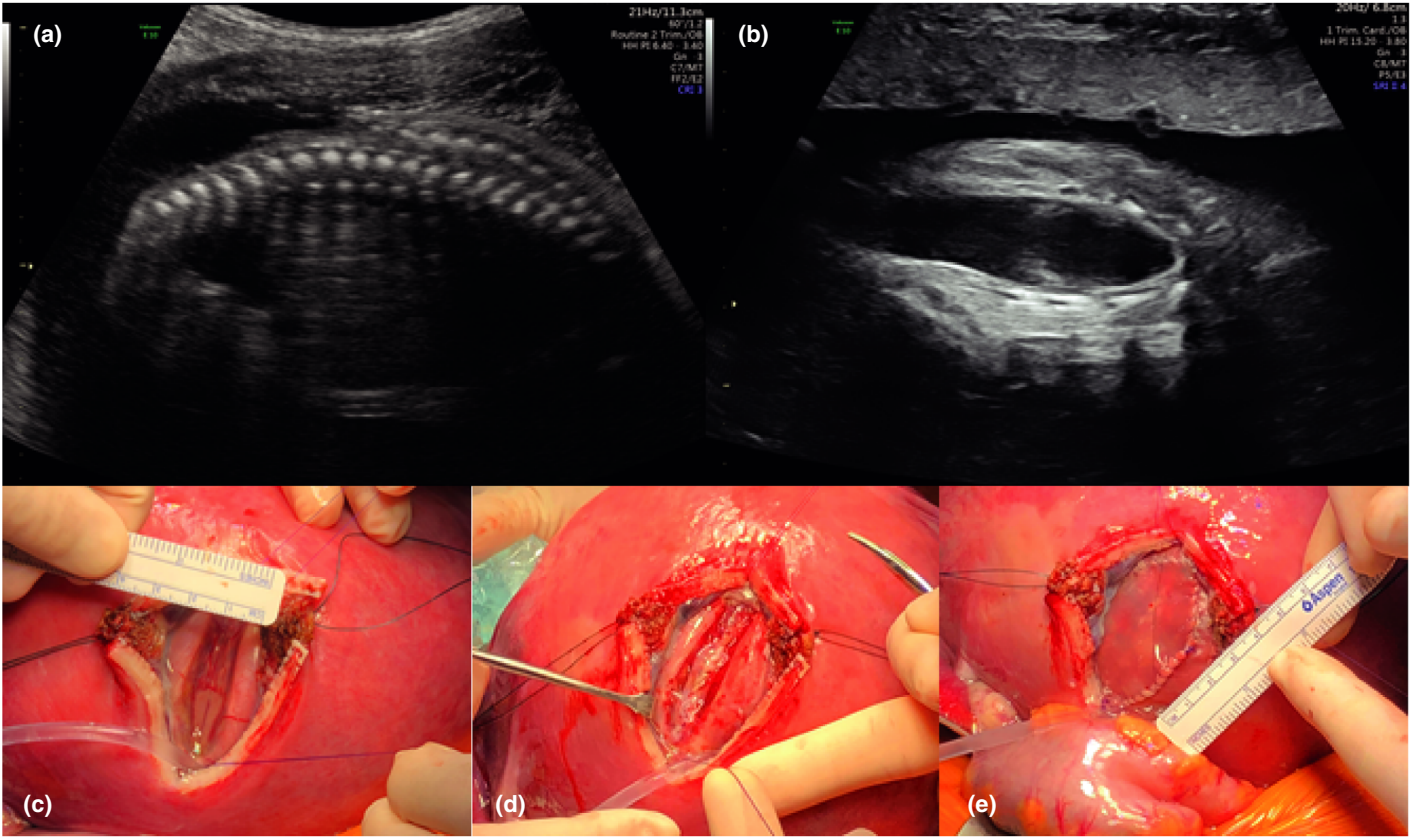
A case of myeloschisis. (a,b) Preoperative ultrasound findings of a relatively wide open lesion; (c) intraoperative images of the lesion prior to repair; (d) following closure of the dura and (e) application of a skin patch. Reproduced with permission from University College London Hospitals, London and Universitair Ziekenhuis Leuven, Belgium.
